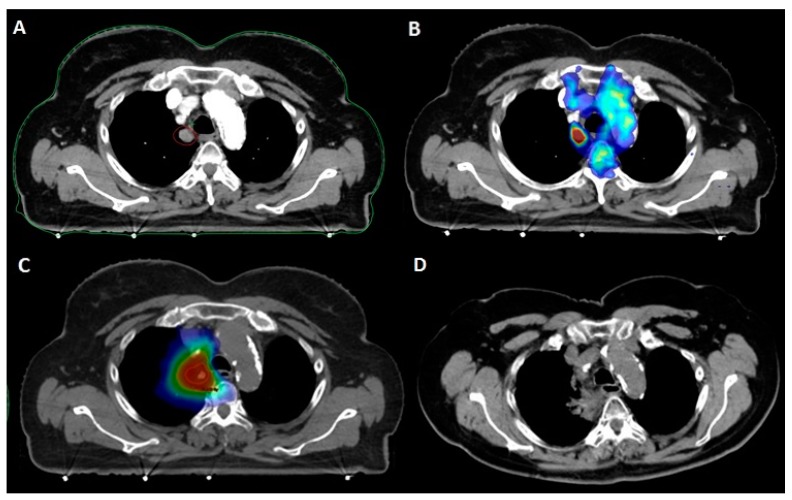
Patient with a clinical history of pT2N0 lung adenocarcinoma treated with upper lobe lobectomy. A: Paramediastinal middle lobe metastasis (12 × 13 mm) detected at follow-up contrast-enhanced chest CT. B: 18FDG-PET/CT fusion showing isolated hypermetabolism of the paramediastinal metastasis. C: SBRT delivering 50 Gy in five fractions to the PTV (dark blue: 20 Gy; light blue: 30 Gy; green: 40 Gy; yellow: 45 Gy; red: 50 Gy). D: CT evaluation at nine months showing radiation fibrosis following complete metabolic response.
